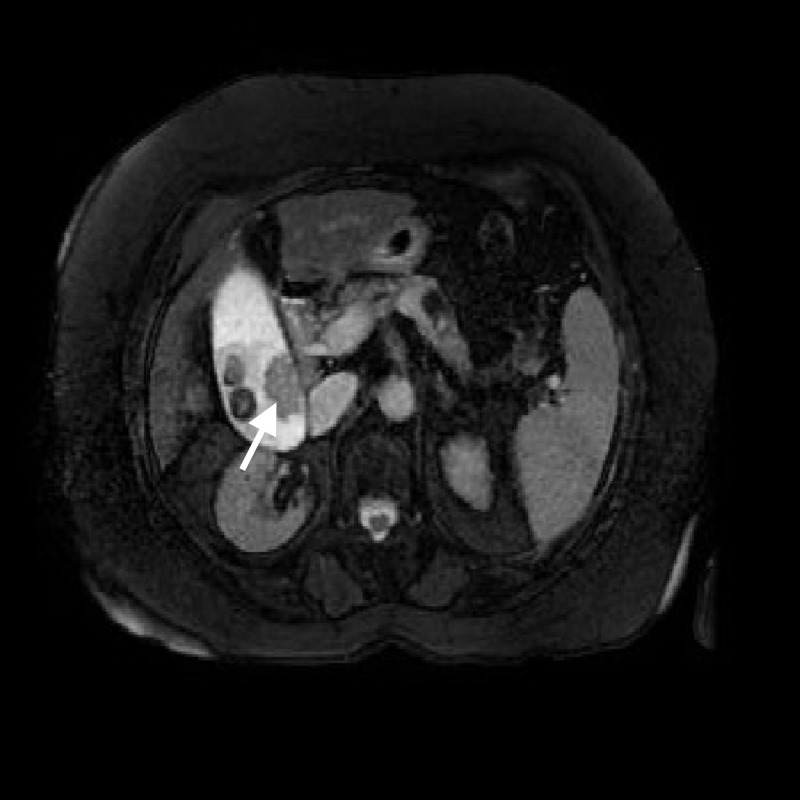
Abdominal computed tomography scan demonstrating a 2.7 x 1.8 cm soft tissue polypoid mass within the gallbladder
